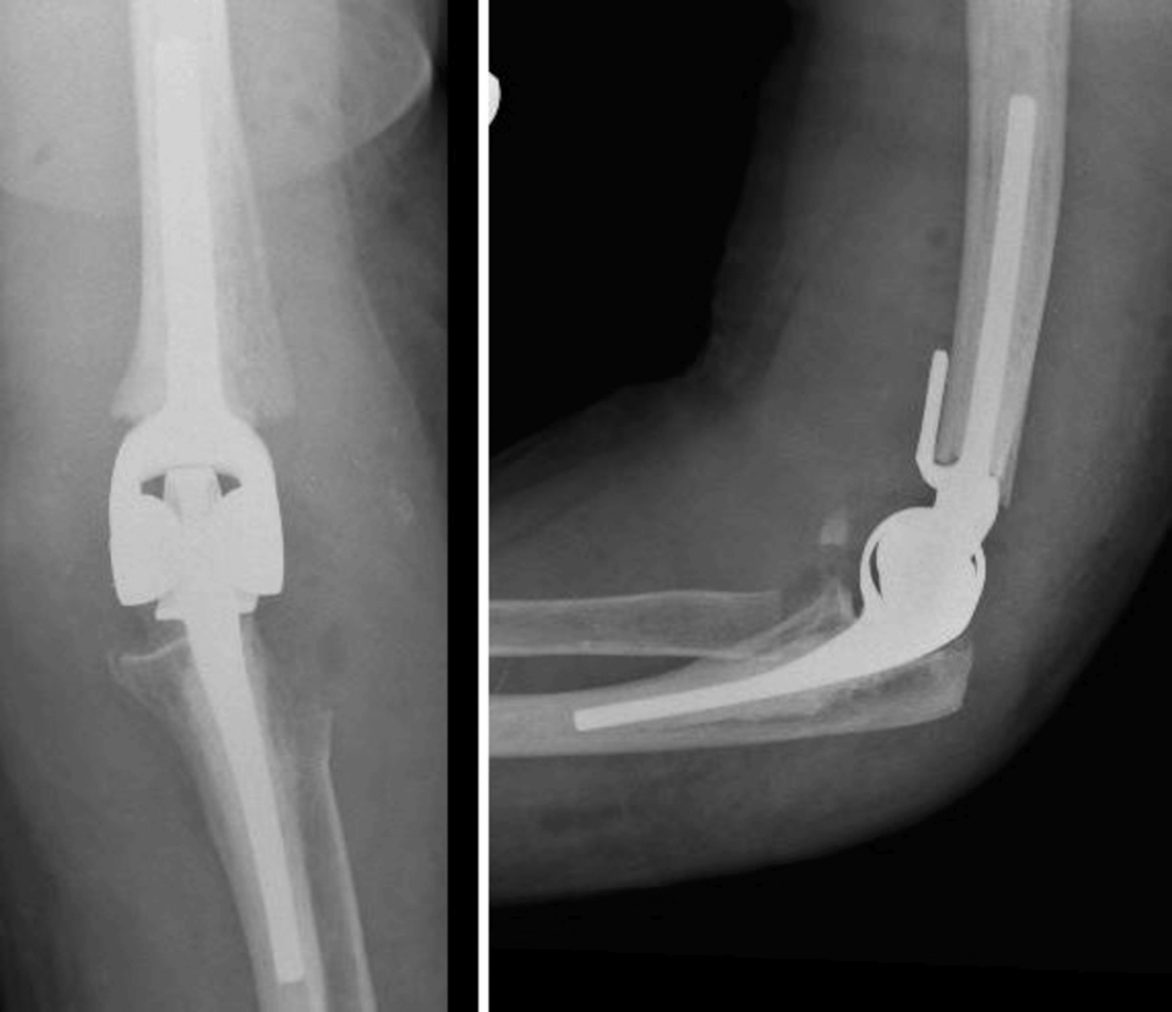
Postoperative radiograph of a patient's elbow after TER. TER: total elbow arthroplasty
